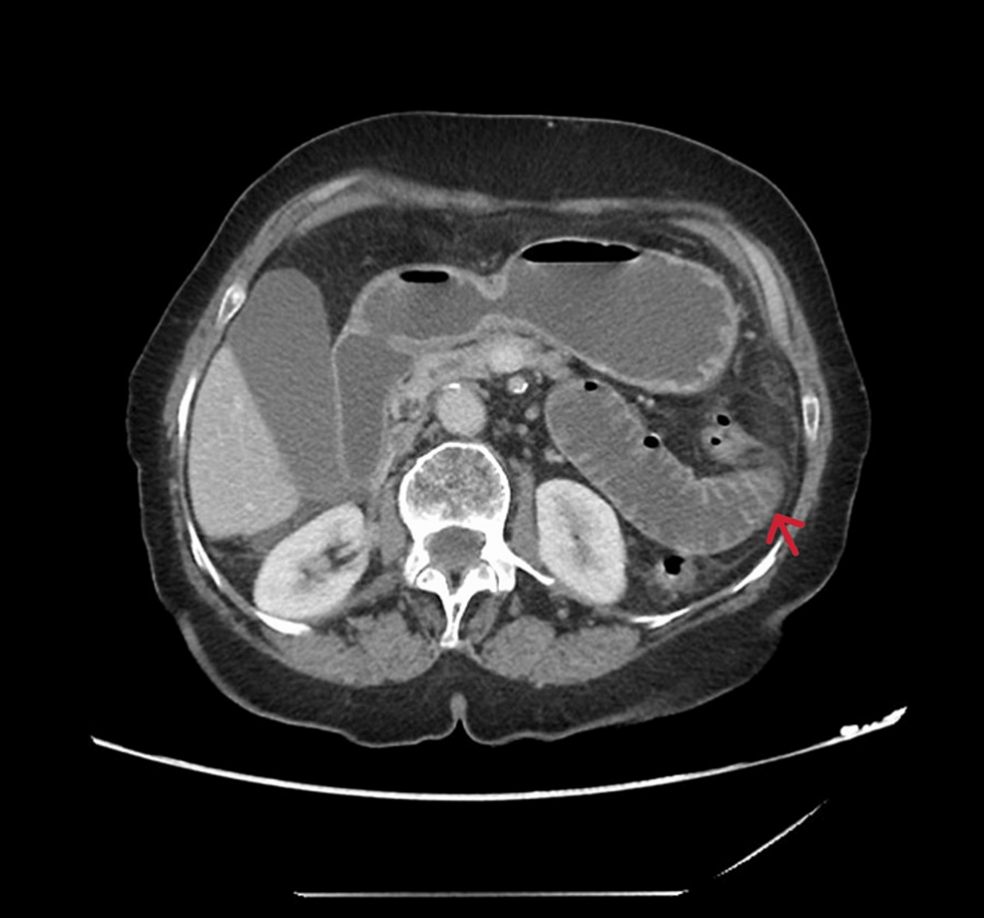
Abdominal CT in axial view showing focal obstruction with the transitional zone at the distal jejunal loop (red arrow) CT: computed tomography
